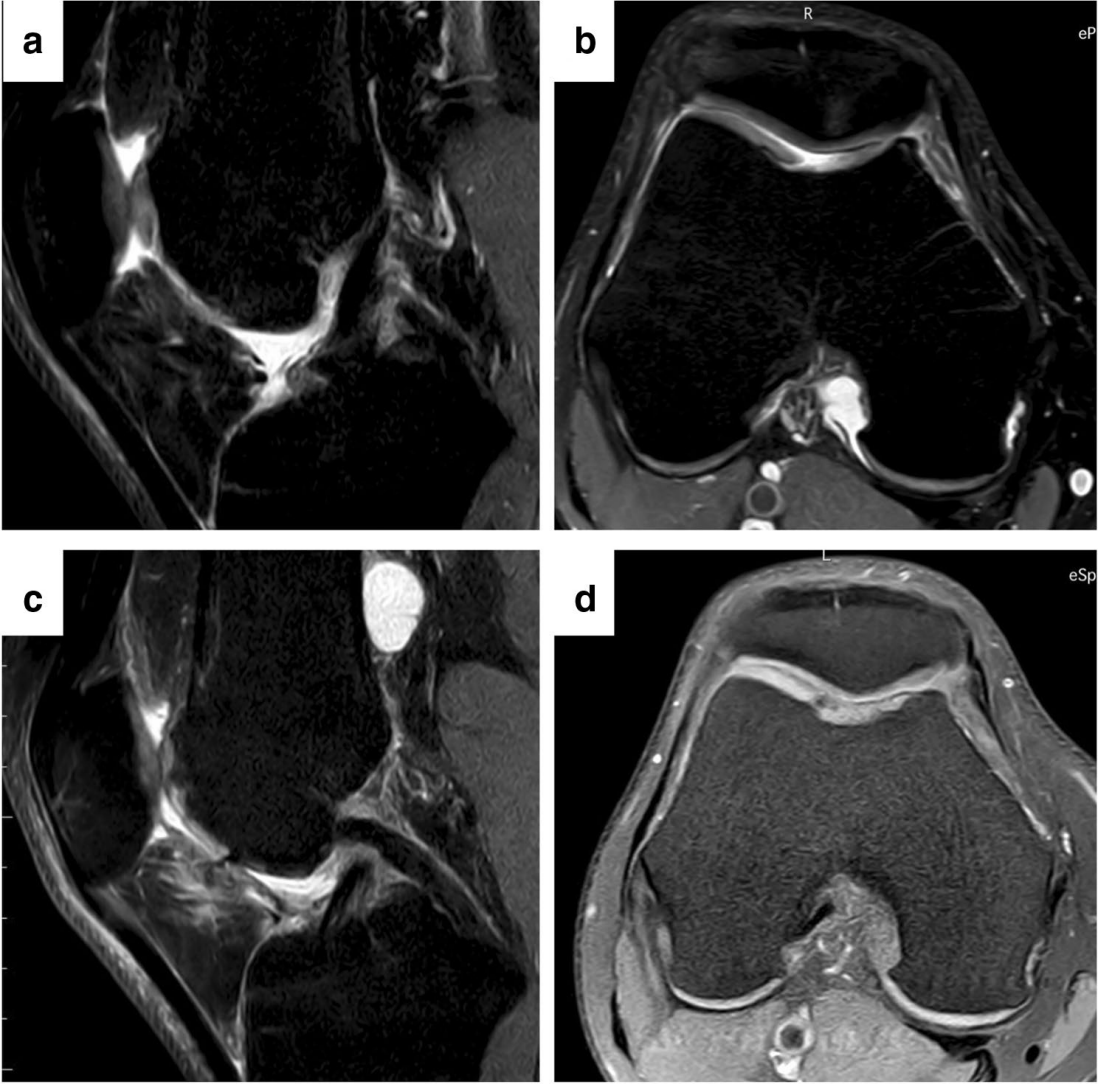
Successful treatment of a grade IV cartilage lesion in the trochlea in early degenerative knee joint environment; a, b preoperative MRI; c, d 1 year postoperative MRI with complete cartilage defect filling
